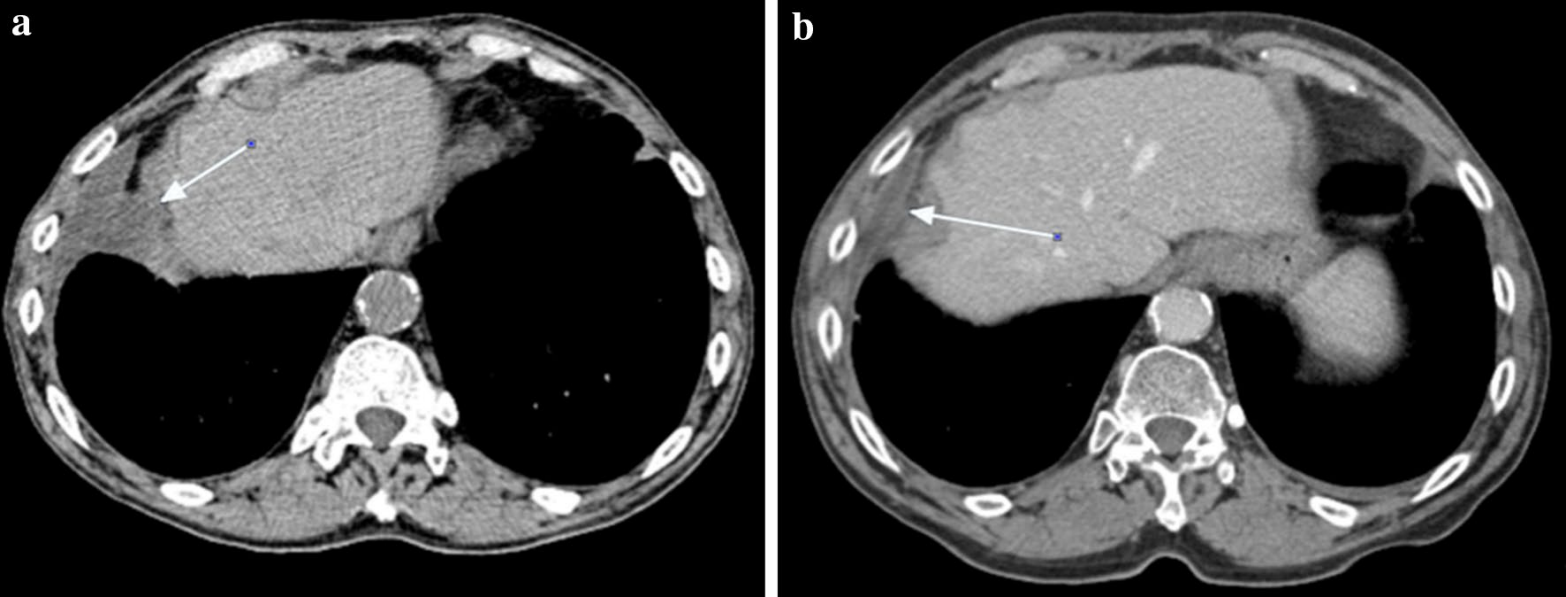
Computed tomography (CT) of the torax. a Pathological tissue with elongated morphology of maximum diameter of 6 cm. b Almost complete disappearance of the pathological mass previously observed
